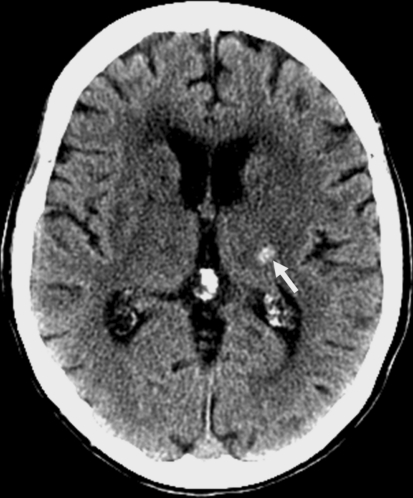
Axial noncontrast computed tomography of the brain revealed an acute, rounded hyperdense lesion in the region of the left thalamus and likely abutting the posterior limb of the internal capsule (*white arrow*), corresponding to a small acute hemorrhage
